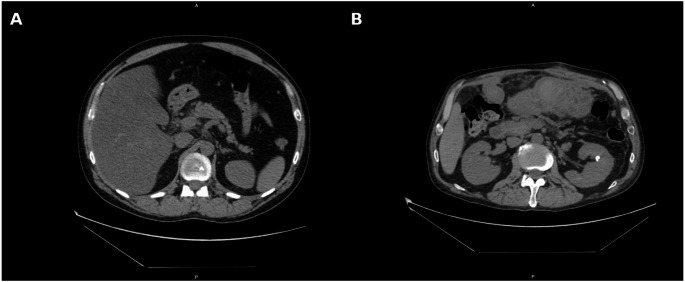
Non-contrast CT morphology in acute pancreatitis. (A) Axial non-contrast CT image showing pancreatic enlargement and peripancreatic inflammatory changes corresponding to Balthazar grade C. (B) Axial non-contrast CT image demonstrating extensive peripancreatic inflammatory changes and fluid collections consistent with Balthazar grade E
